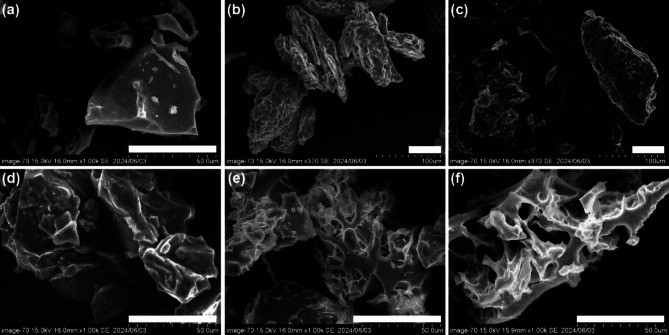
Morphological attributes of rice starch granules and supplements. (a) Normal cooked rice (CT); (b) dried chili pepper powder; (c) dried wasabi powder; (d) cooked rice added with rapeseed oil (RRS); (e) cooked rice added with dried chili pepper powder (CRS); (f) cooked rice added with dried wasabi powder (WRS), scale bars indicate 50 μm.
